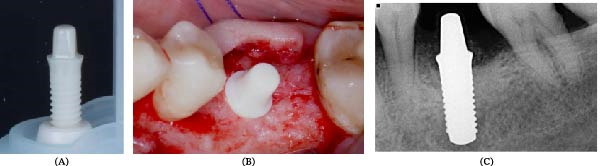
(A) Conventional zirconia implant before placement. (B) An intraoperative photograph showing implant insertion into the prepared osteotomy site; measurement markers on the periodontal probe reflect spatial orientation. (C) Postoperative periapical radiograph acquired using a standardized long‐cone paralleling technique, with millimeter calibration for evaluation of implant seating and crestal bone levels.
